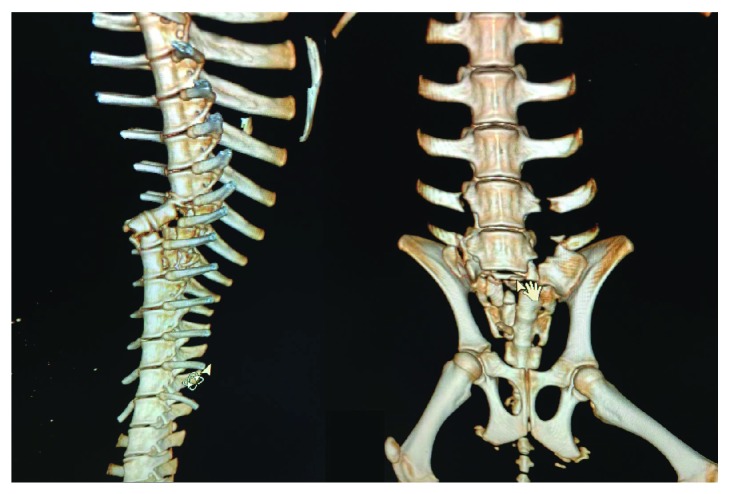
3D CT reconstruction of the spine and pelvic fractures.
